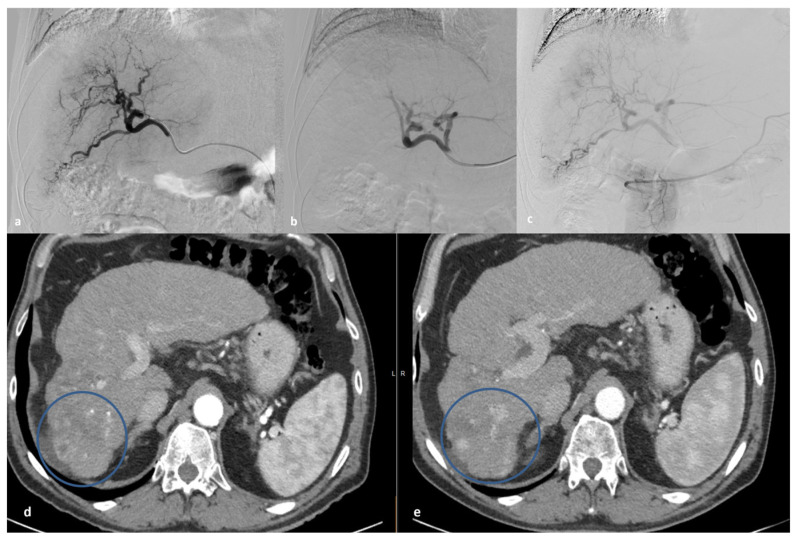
A 63-year-old cirrhotic patient with multifocal right lobe HCC who underwent 3 sessions of doxorubicin DMS-TACE. (a) Digital subtraction angiography (DSA) from the right hepatic artery depicting the arterial vasculature and the HCC hypervascular lesions of the right hepatic lobe. (b) Final DSA following the 1st session of whole-lobe TACE using 100 mg of doxorubicin and degradable starch microspheres, demonstrating complete devascularization of the right hepatic lobe. (c) Initial DSA prior to the 2nd DMS-TACE session (1 month after the 1st session), demonstrating complete viability of the right-lobe arterial supply, including the feeding vessels of the target HCC lesions. (d) A 1-year follow-up CT (arterial phase) demonstrating sustained complete response of the treated lesions (circle), compared to preprocedural imaging (e), while aFP levels remain decreased, at normal values.
